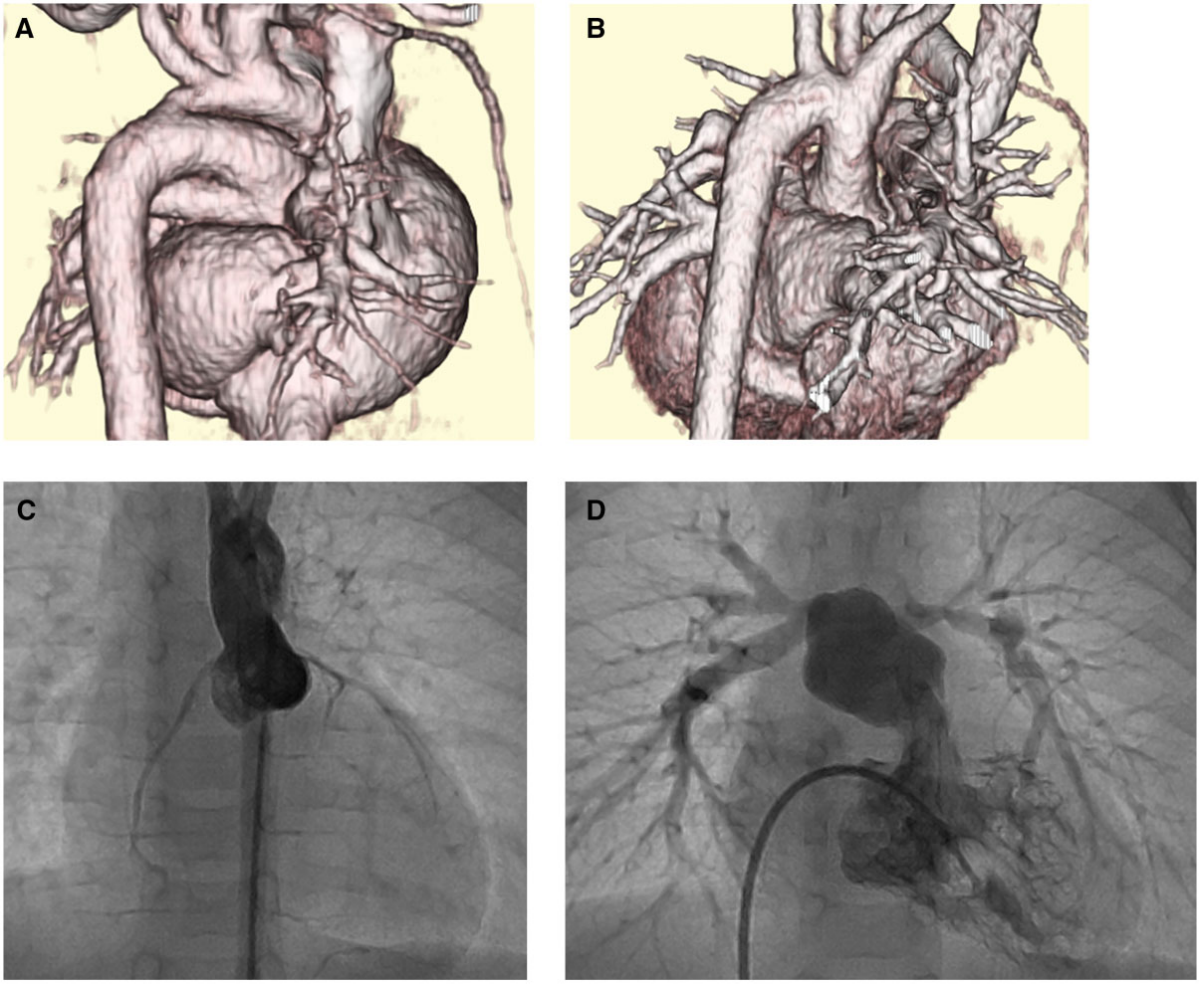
Computed tomography and cardiac catheterization. (A) Preoperative: coarctation of the aorta with hypoplastic aortic arch. (B) Postoperative: no re-coarctation of the aorta. (C) Postoperative aortic angiography shows intact coronary artery ostium and no aortic valve regurgitation. (D) Postoperative right ventriculography shows intact right ventricular outflow tract.
